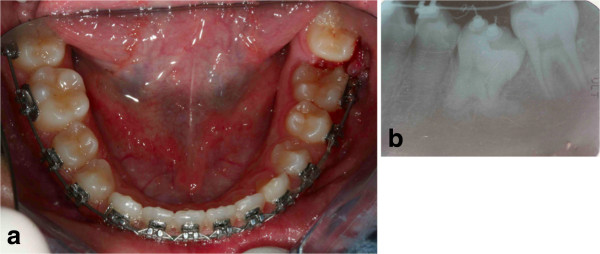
After 6 months. (a) Intraoral photo. (b) Intraoral radiograph. Pre-informed brackets and straight archwires were used; approximately 6 months after the beginning of the fixed treatment, the tooth appeared in the dental arch.
